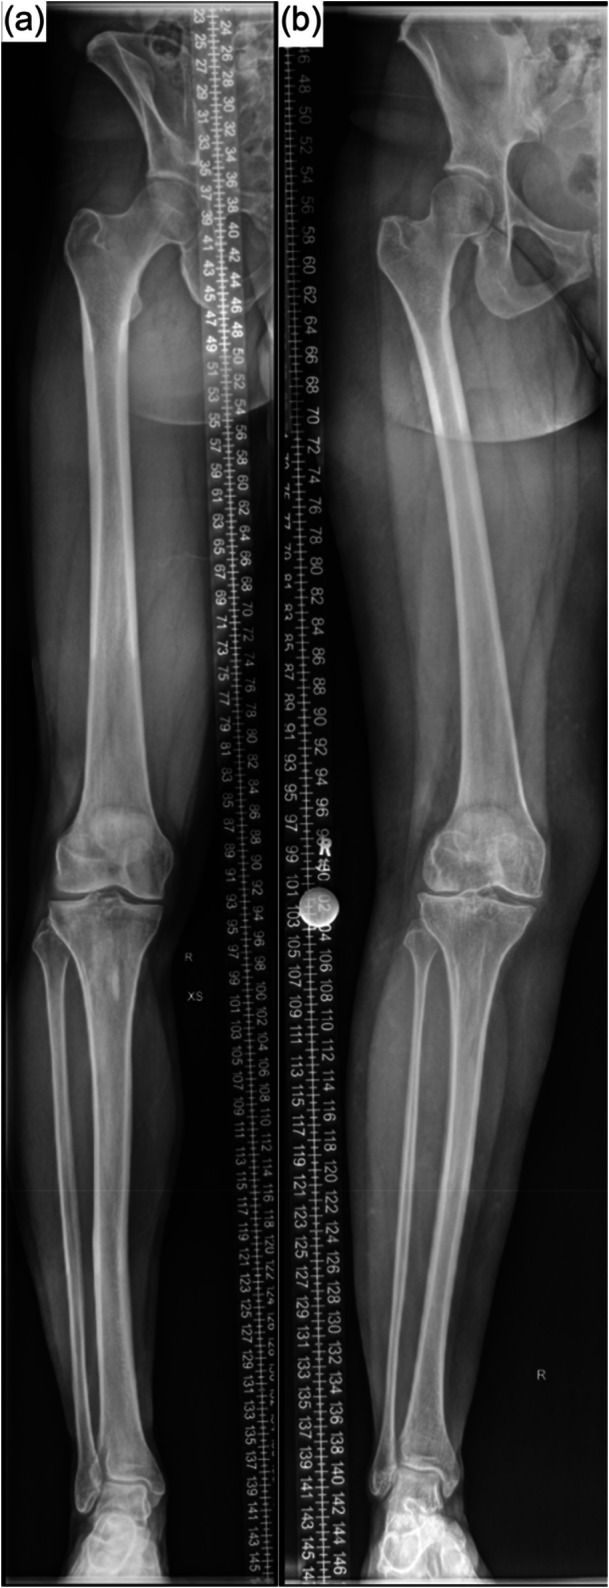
Preoperative whole leg radiographs taken in the knee forward position. (a) A preoperative genu varum while (b) shows a genu valgum.
